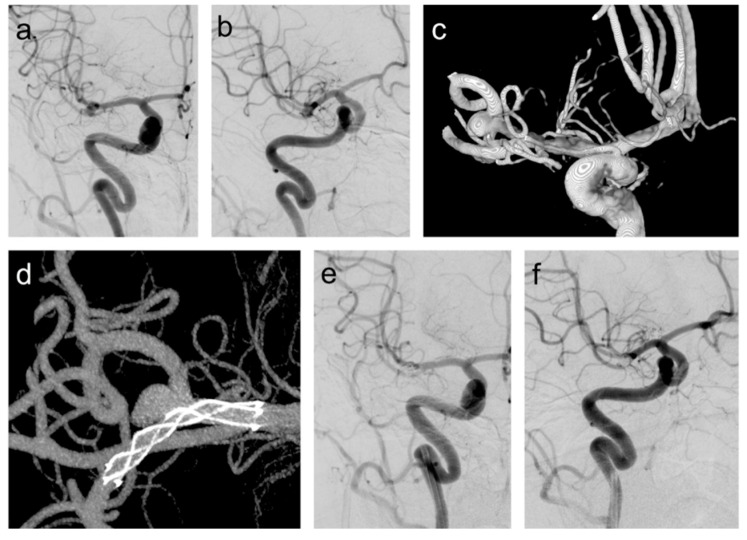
(a,b) A patient with a wide-necked right middle cerebral artery (MCA) bifurcation aneurysm. The aneurysm was treated with the stent-assisted coiling using the low-profile visualized intraluminal support junior (LVIS Jr.); (c) three-dimensional reconstruction demonstrates the complex morphology of the aneurysm; (d) intraprocedural contrast-enhanced flat panel detector computed tomography (CT) (maximum intensity projection (MIP) reconstruction) showing full stent opening with wall adherence along the length of the device; (e,f) Post-procedural digital subtraction angiography (DSA) showing complete occlusion of the aneurysm.
